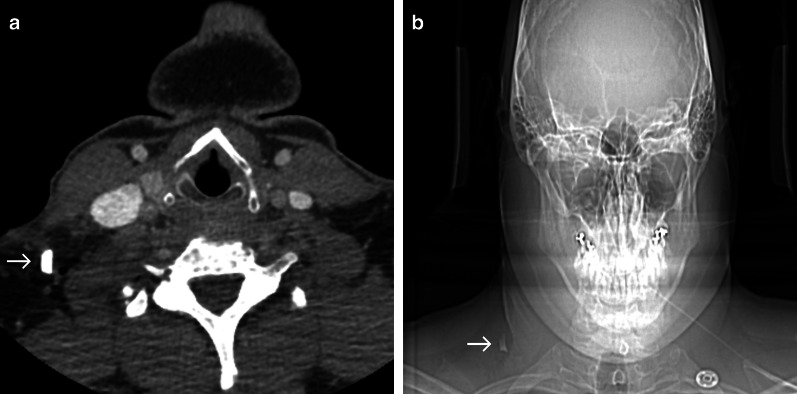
Glass bottle fragment. Axial contrast enhanced CT image (a) and corresponding anteroposterior scout scan (b) displaying a hyperdense structure (arrow) in the right posterior cervical space dorsolateral to the sternocleidomastoid muscle. At first glance, this object could look like a metal fragment (compare Fig. 7b). Careful comparison, however, reveals a lack of streak artefacts in CT images which would be expected in metallic objects of this size
